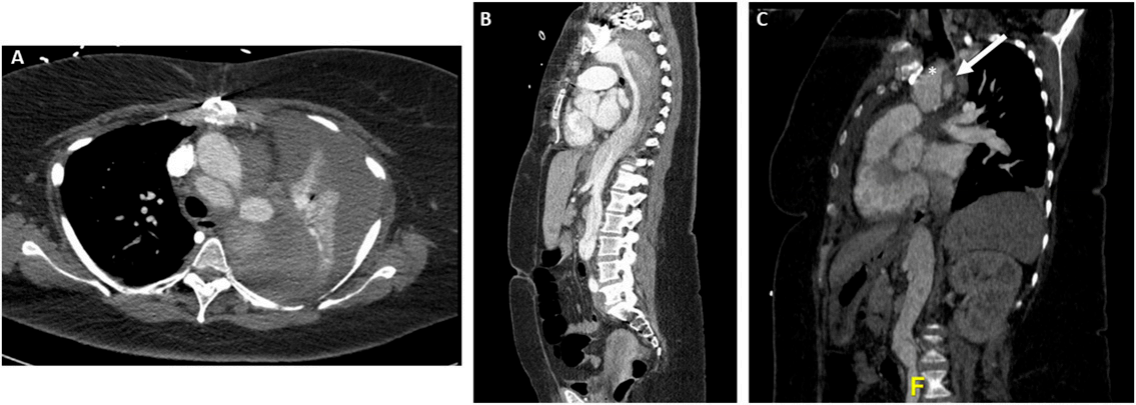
Axial (A) and sagittal (B) preoperative computed tomography angiogram (CTA) with massive left hemothorax and dissected aneurysm extending from the descending thoracic aorta to the right common iliac artery. (C) Close-up sagittal cut with demonstration of the dissection flap (arrow) and innominate artery origin (asterisk).
